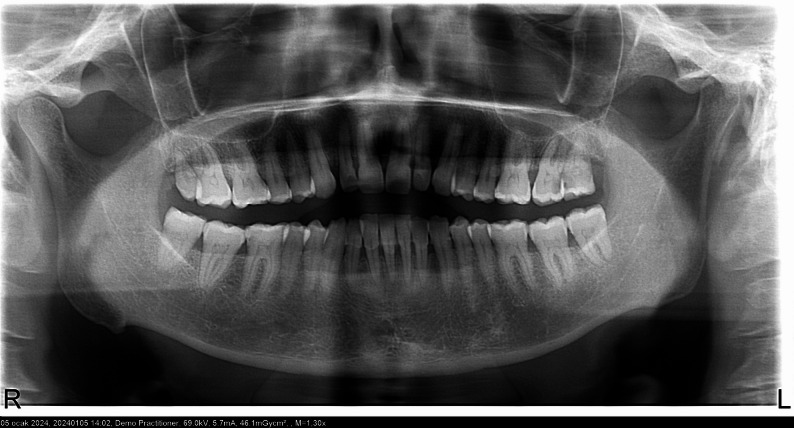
Panoramic radiograph of a patient with diabetes mellitus showing pulp stones in the molar region
